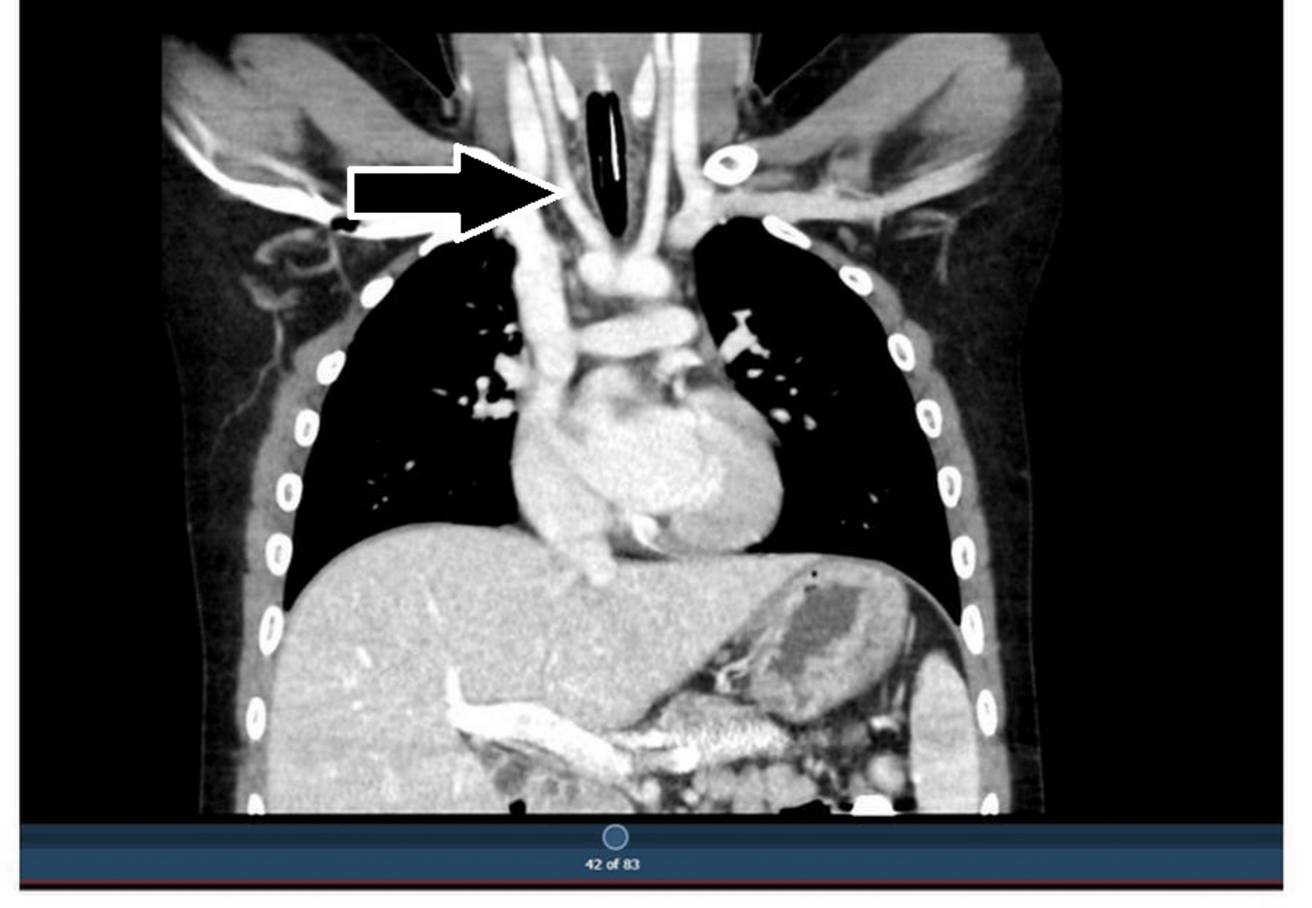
Coronal CT angiography showing a double aortic arch encircling the trachea and esophagus, leading to posterior esophageal compression
